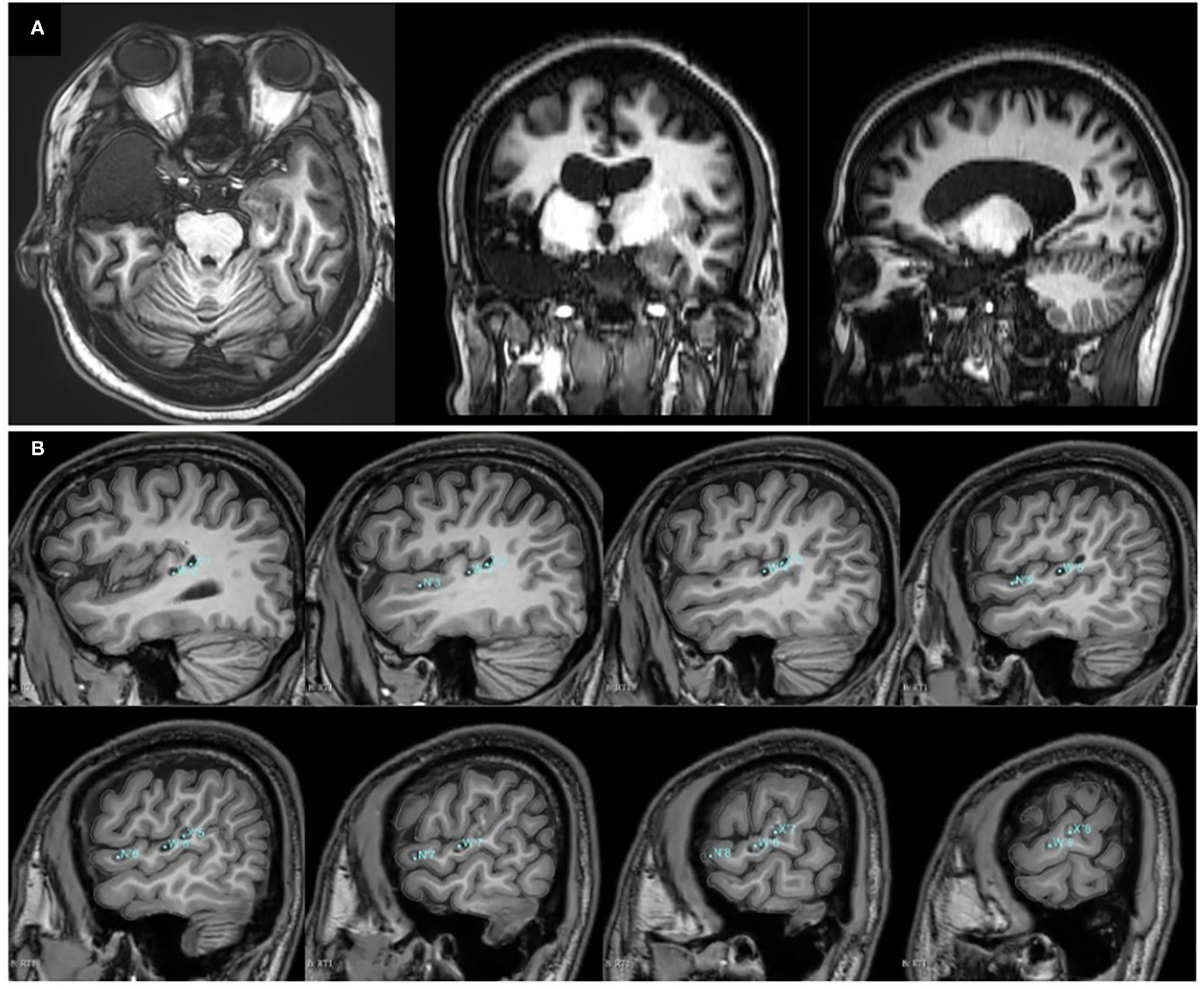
Postoperative T1-weighted MRI results. (A) T1-weighted MRI results of case 1 after the right anteromedial temporal lobe and insular lobe resection. (B) T1-weighted MRI results of case 2 after SEEG-guided radiofrequency thermocoagulation (RFTC). The locations and labels of the electrodes for the thermocoagulation were marked in blue.
